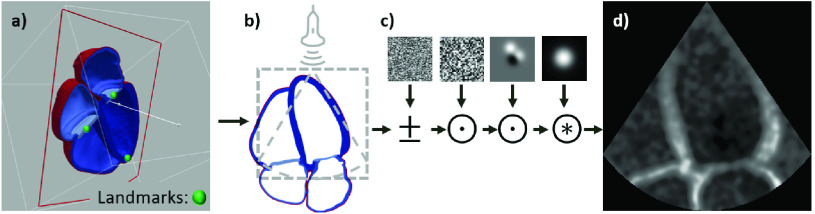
Extraction details for echocardiography. To extract pseudo images a) a 2D plane is defined from a set of landmarks, b) the plane is rotated and cropped to match standard acquisition parameters and positioned to match standard positioning in real images, c) random noise and shadows are added and the slice is blurred yielding d) the final pseudo image. Additional details are given in Appendix A.
